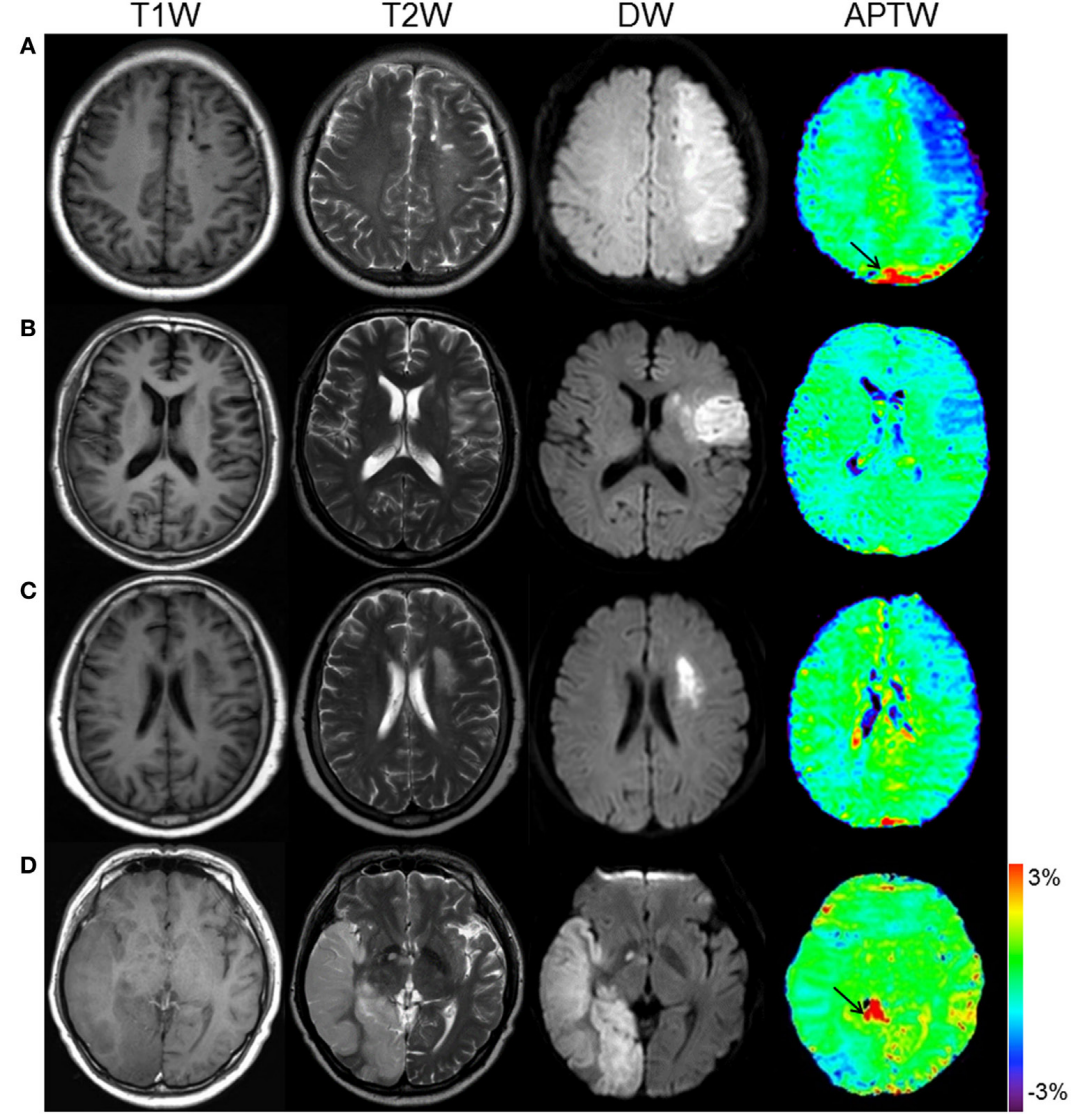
APT and conventional MR images for different stage patients. (A) F/50 years, a hyperacute stage patient at 2 h after symptom onset. APTW_ave_ = −0.83%, APTW_max–min_ = 1.30%. (B) M/54 years, an acute stage patient at 17 h after symptom onset. APTW_ave_ = −0.63%, APTW_max–min_ = 0.99%. (C) F/62 years, an early subacute stage patient at 72 h after symptom onset. APTW_ave_ = −0.40%, APTW_max–min_ = 0.72%. (D) M/54 years, a late subacute stage patient at 120 h after symptom onset. APTW_ave_ = 0.03%, APTW_max–min_ = 0.53%. Note the presence of CSF artifacts (black thin arrows).
